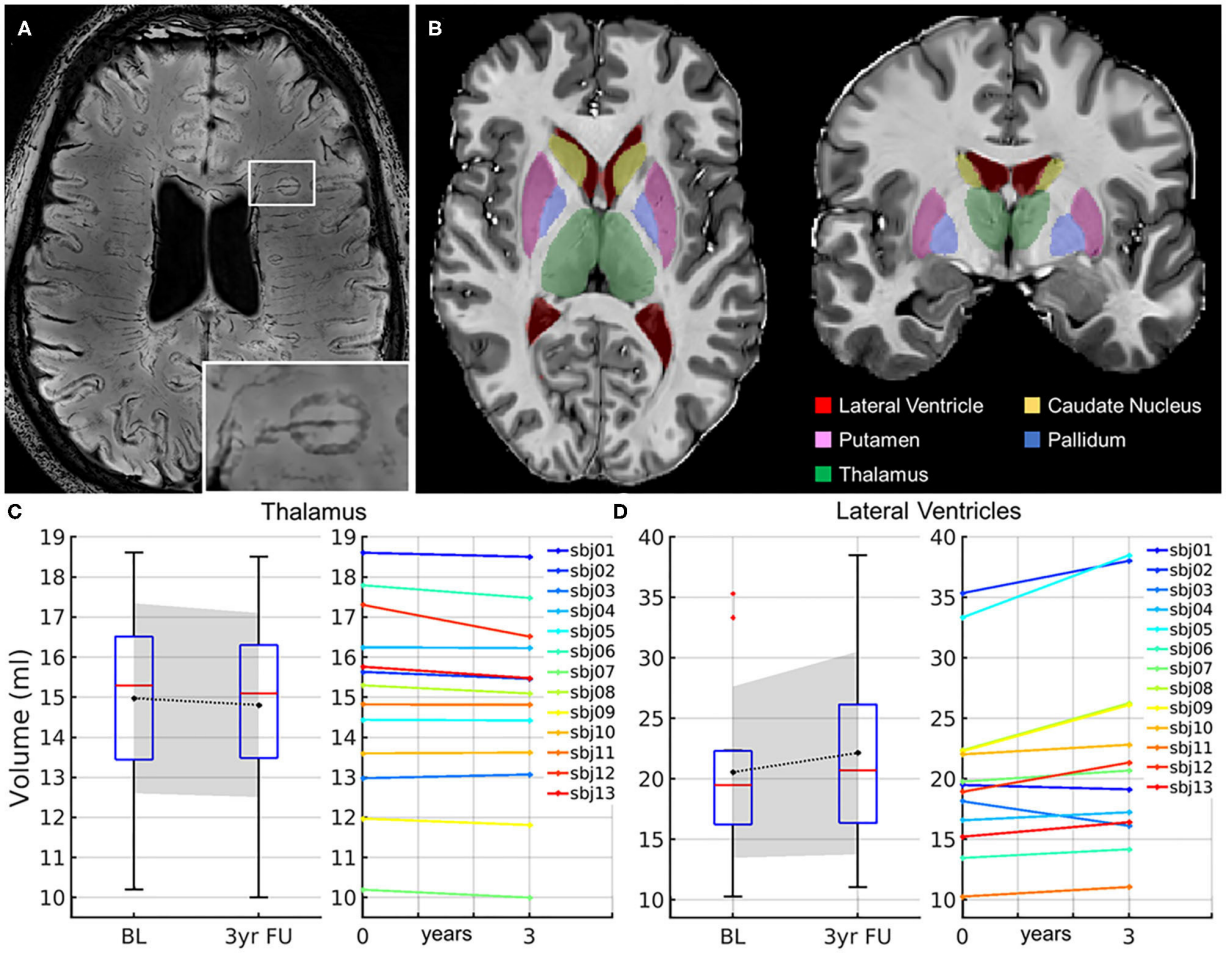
Images scanned at 7T. (A) FLAIR-SWI overview image shows multiple hyperintense white matter lesions surrounded by SWI-detected hypointense iron rims in a 27-year-old male relapsing-remitting multiple sclerosis (RRMS) patient with 7 years of disease duration and a Multiple Sclerosis Severity Score (MSSS) of 6.98. The white rectangle indicates the magnified iron rim lesion (IRL). (B) Segmentation of the subcortical deep gray matter structures. Subcortical segmentation maps are overlaid on the 7T T1-weighted MP2RAGE single-subject template from a male RRMS patient. (C) Significant reduction of the thalamus volume within 3 years within the cohort (left) and according to individual courses (right). (D) Significant enlargement of the lateral ventricles of the cohort (left) and according to individual courses (right). BL, baseline; FU, follow-up; ml, milliliter; sbj, subject; yr, year.
